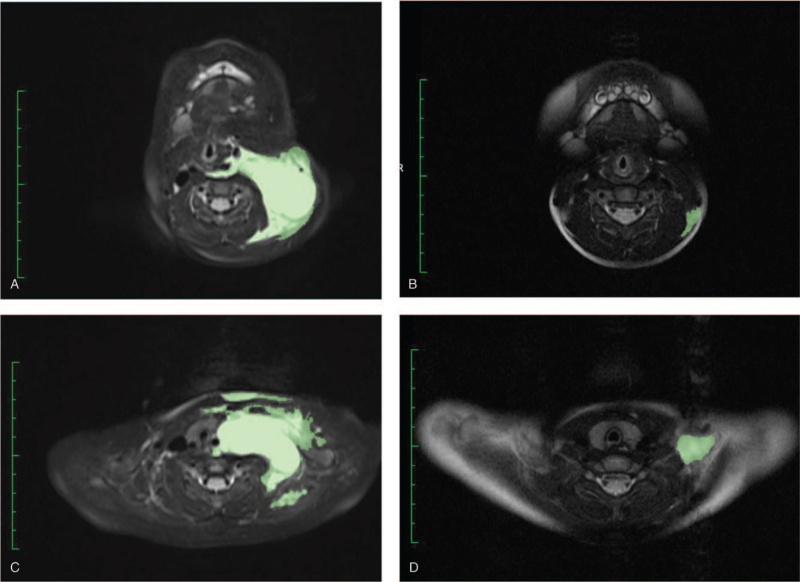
MRI of volumetric measurements for Case 4 (8-month-old woman with neck and mediastinal lymphatic malformation). T2-weighted MRI of the chest and neck demonstrates cystic lymphatic malformation, which is the target lesion. (A, C) Pretreatment; (B, D) 15 months after the administration of eppikajyutsuto. The volumetric measurements evaluated using the Digital Imaging and Communications in Medicine viewer (OsiriX) are also shown. MRI = magnetic resonance imaging.
